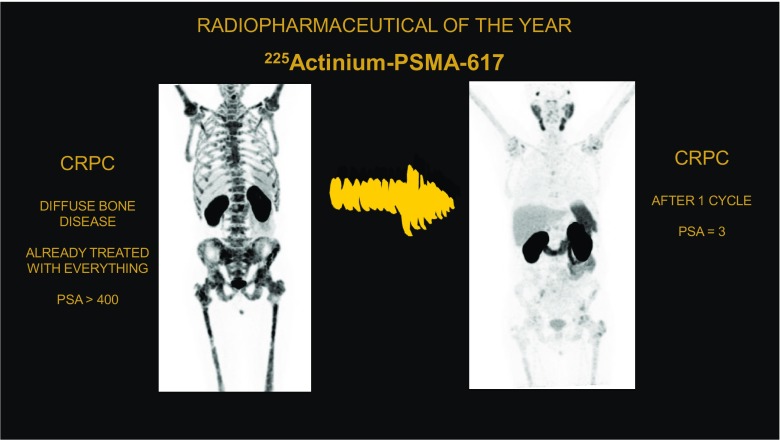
An impressive case of treatment response after only one cycle of ^225^Ac-PSMA 617, the radiopharmaceutical of the year at EANM 2017. Data on therapeutic efficacy [48]
